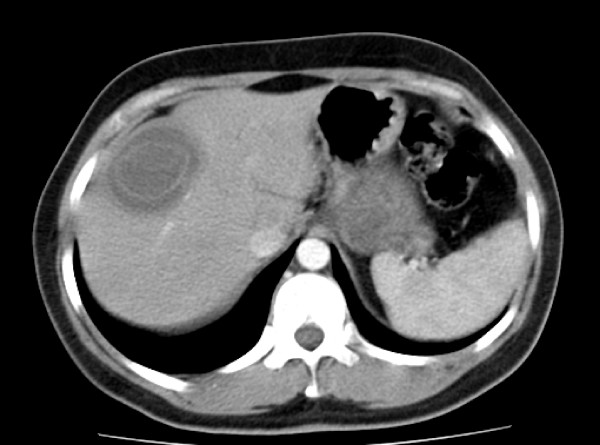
2 Computed tomographic appearance of the echinococcal cyst in hepatic segment VIII.
